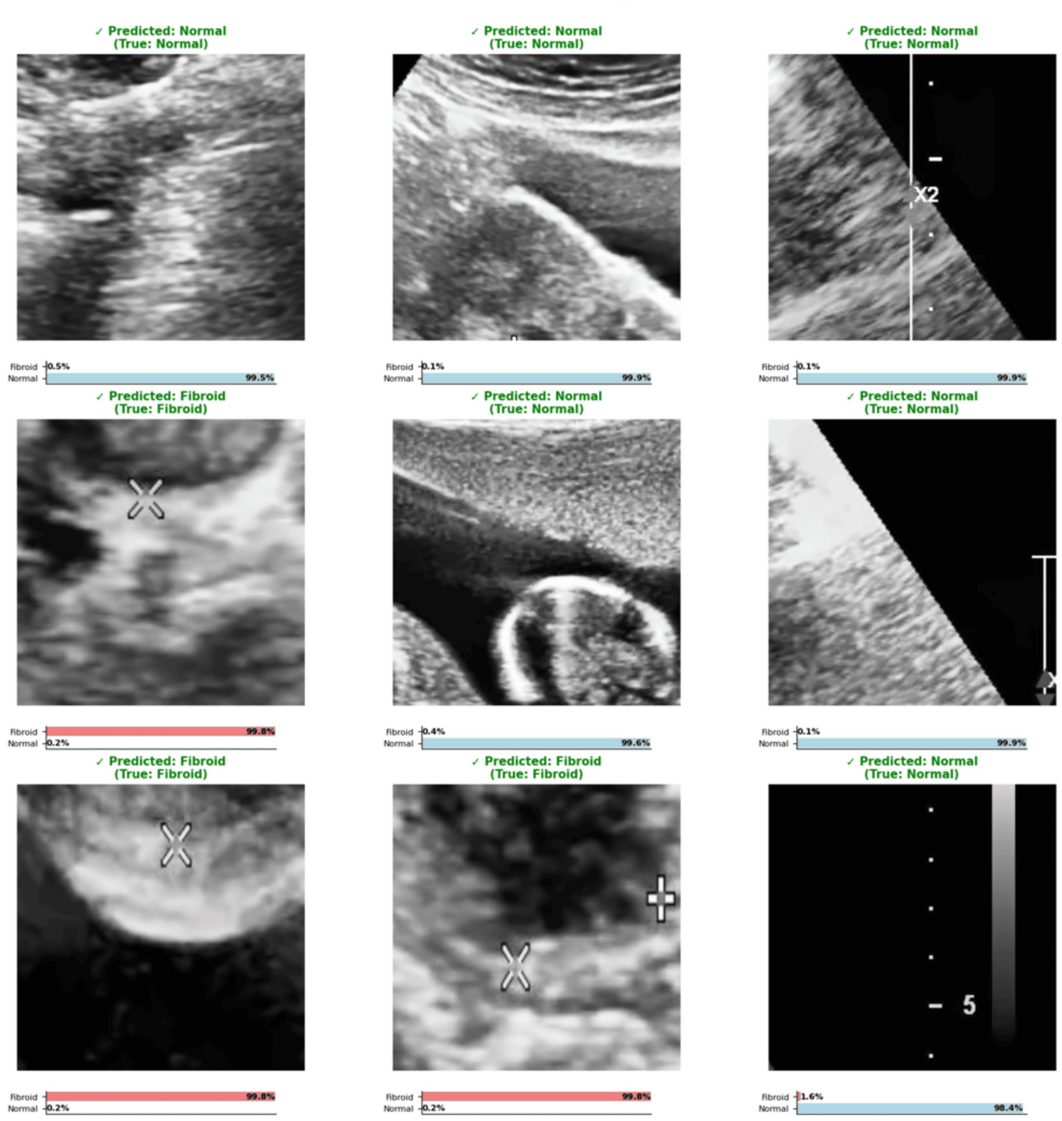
HiFi-ViT prediction results on raw validation images Representative predictions from the HiFi-ViT model on validation set ultrasound images, showing predicted class labels (fibroid/normal) with confidence scores. Images are from the publicly available uterine fibroid ultrasound dataset [17]. The model demonstrates high confidence in correct predictions, with fibroid cases showing >99% confidence and normal cases showing >98% confidence. HiFi-ViT: Hierarchical Fibroid Vision Transformer
